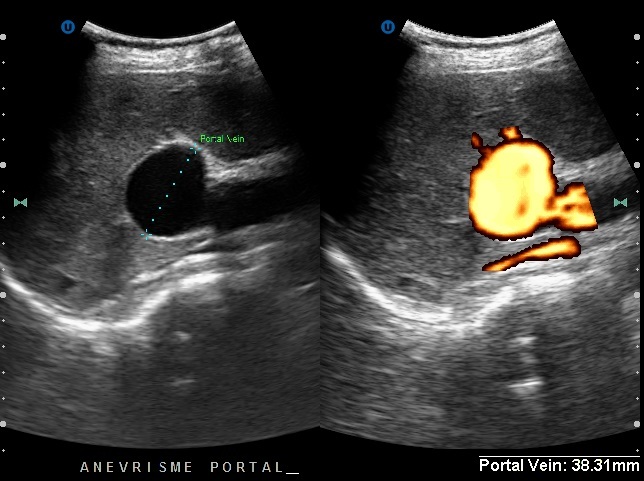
Portal vein presenting a fusiform dilatation in ultrasonography
